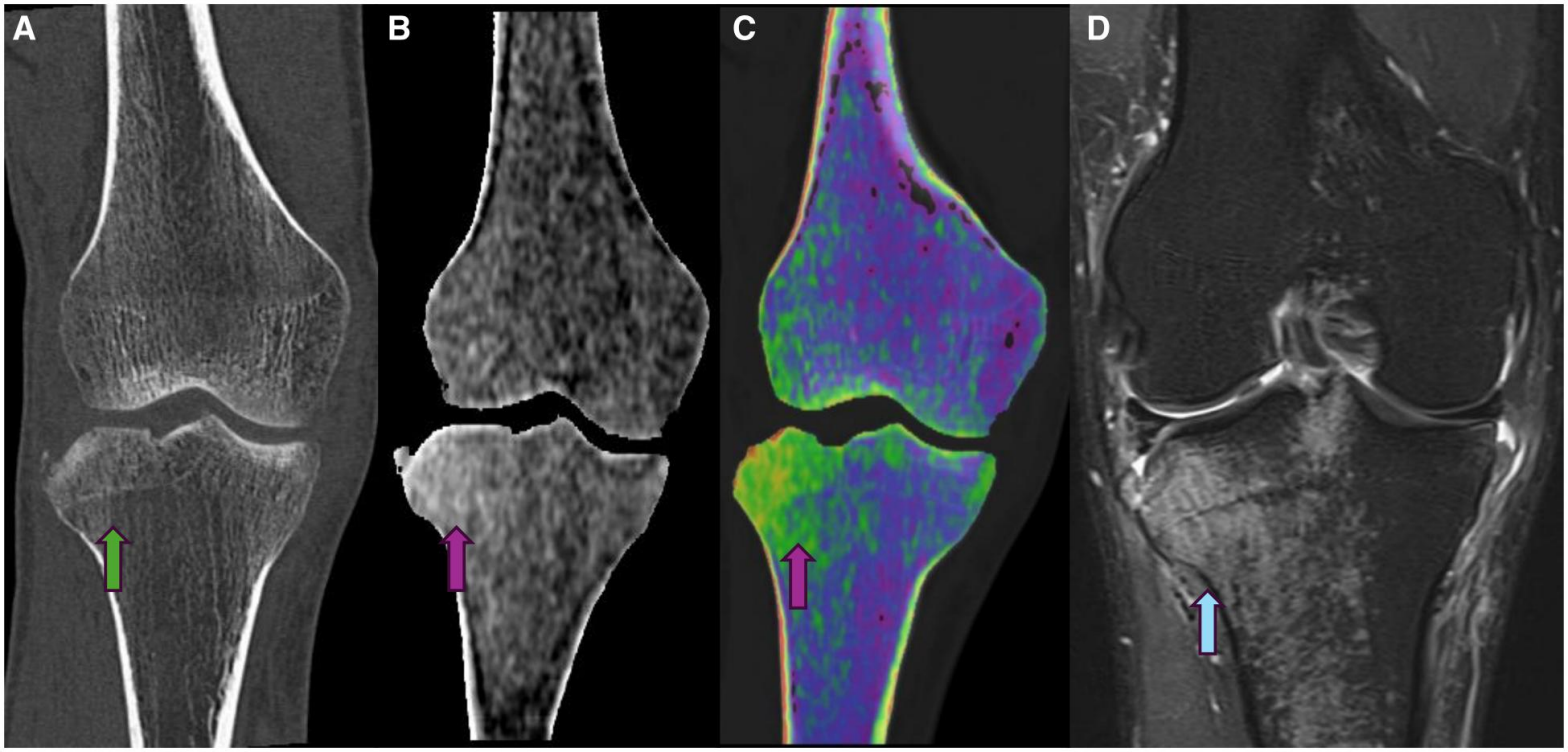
Patient with a history of acute knee injury trauma. A (green arrow) shows routine CT without significant difference of density and a Segond fracture. B and C show marrow oedema on DECT marrow oedema. Findings were confirmed with MRI as shown in D.
